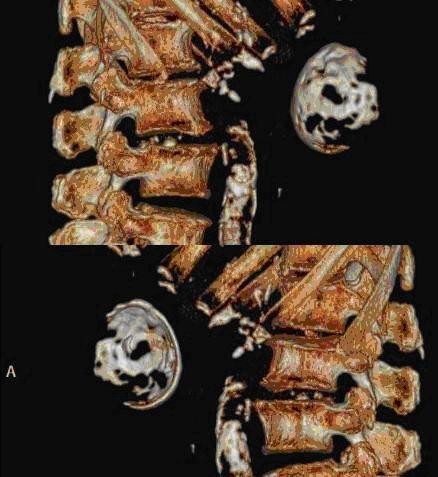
3D CT reconstruction showed an unusual semicircular calcified plate in the upper abdomen. (upper: Right side view, lower Left side view) Ingested foreign body was excluded by history and large size of the calcification.
